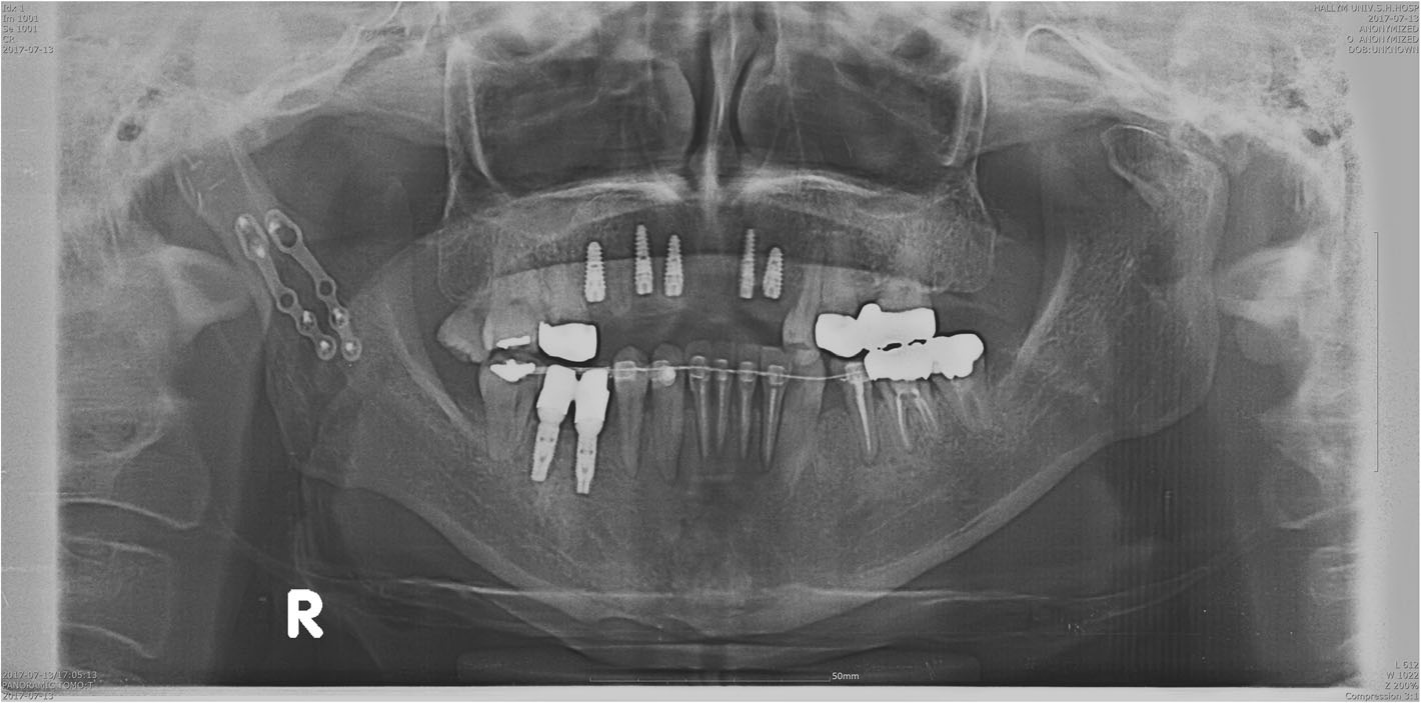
Postoperative panoramic radiographs treated with YK plate
